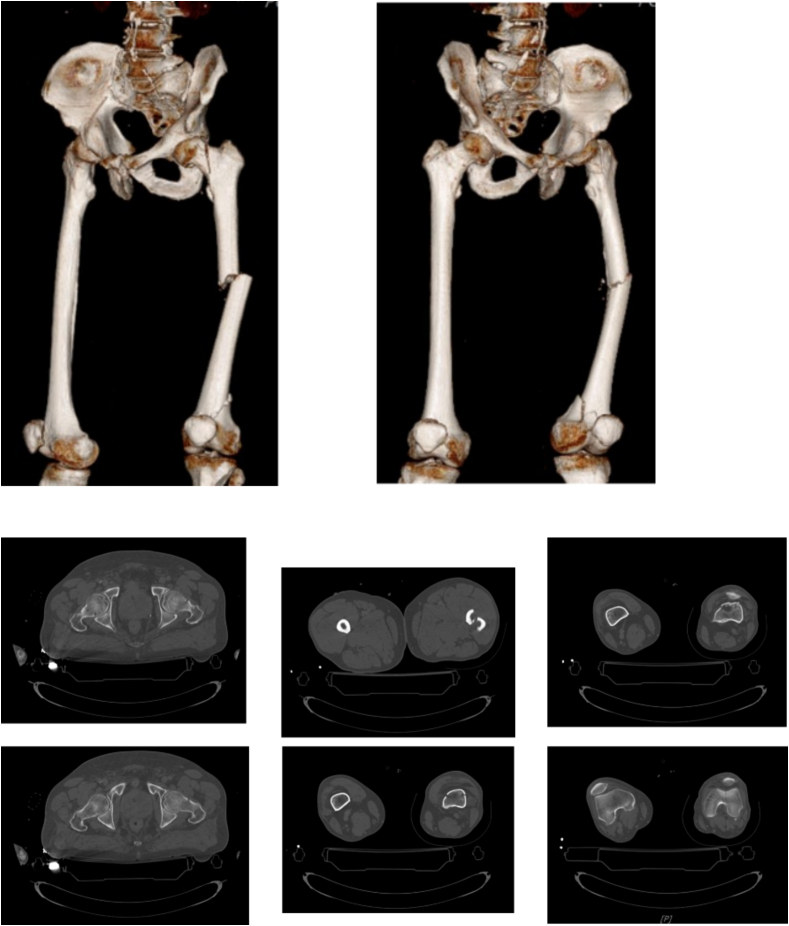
CT at the entrance to the emergency room.
